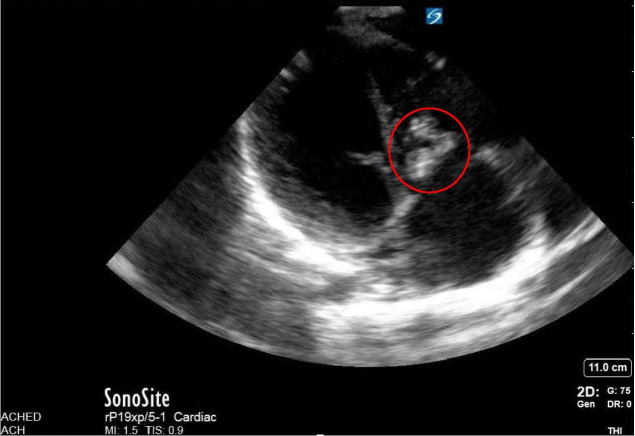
This is a still image of an apical four chamber view of the heart. Overall, there is a thickness noted in one of the leaflets of the tricuspid valve. With his history of fever, suspicion of tricuspid valve endocarditis leading to symptoms of right heart failure was suspected.
